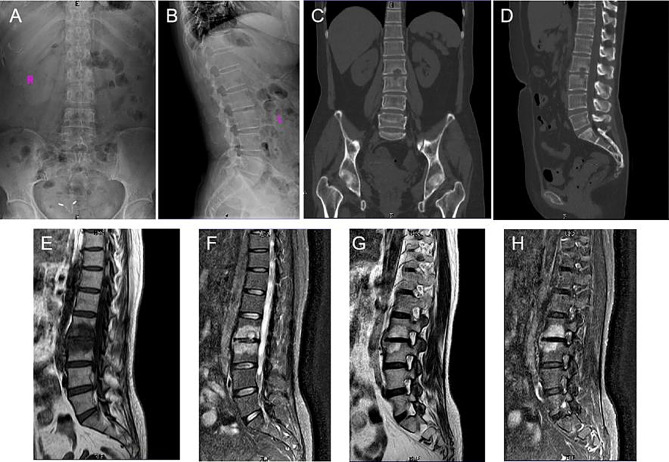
Imaging images of typical cases of brucellar spondylitis. Brucellar spondylitis. A-B: X-ray films show the intervertebral space at L2-L3 is slightly narrowed. C-D: CT images show the intervertebral space between the L2 and L3 vertebral bodies is slightly narrowed, a round-like hypodense shadow is visible within the vertebral bodies, with slightly increased bone density in the surrounding area. The anterior margin of the L2 vertebral body exhibits an irregular morphology, characterized by osteophyte formation and partial discontinuity of the bone cortex. E-H: MRI images show the intervertebral space between the L2 and L3 vertebral bodies is slightly narrowed. Patchy areas of long T1 and slightly long T2 signal intensity are observed within the bone substance at the superior and inferior margins of the L2 and L3 vertebral bodies, which appear hyperintense on the fat-suppression sequence. The L2-L3 intervertebral disc demonstrates heterogeneous signal intensity, with localized slightly hyperintense regions on the fat-suppression sequence.
